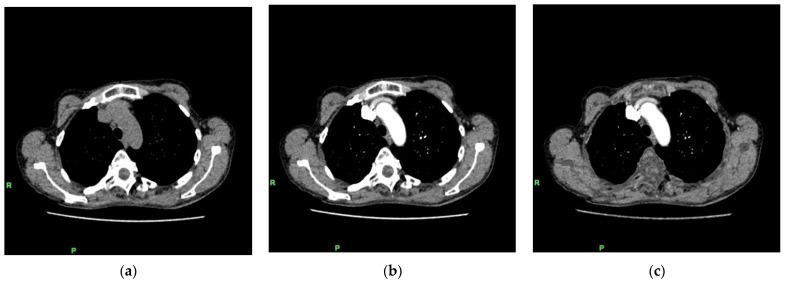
Lung subtraction CT generation process. Noncontrast chest CT axial image (a) and CTPA axial image (b) can be spatially matched based on deformable image registration by subtracting the noncontrast image from the contrast-enhanced image to generate a lung subtraction CT axial image (c). CTPA, CT pulmonary angiography.
